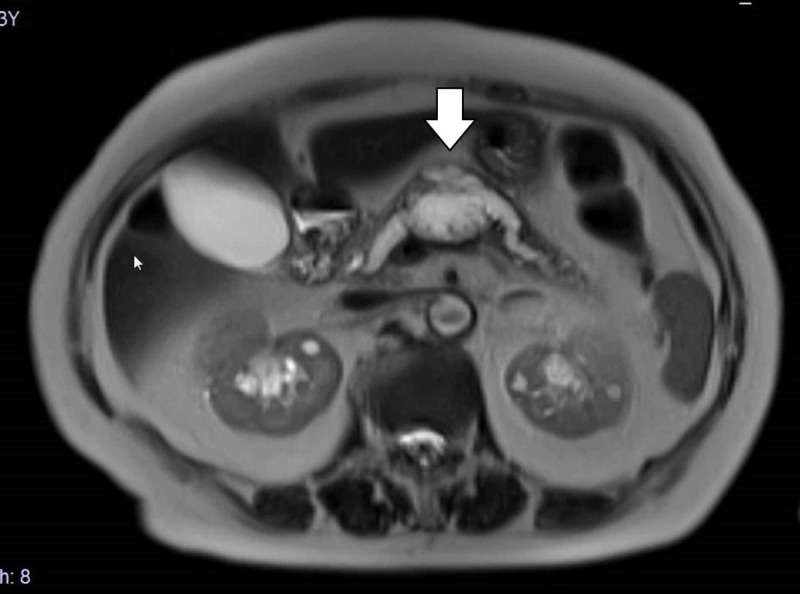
Magnetic resonance imaging T2-weighted magnetic resonance imaging showed the mass as a high intensity area.
